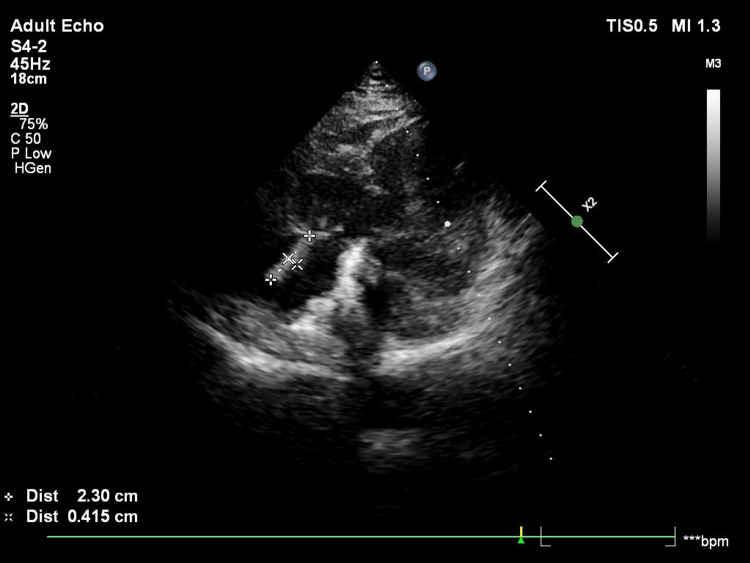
Echocardiogram revealing vegetation in the tricuspid leaflet
